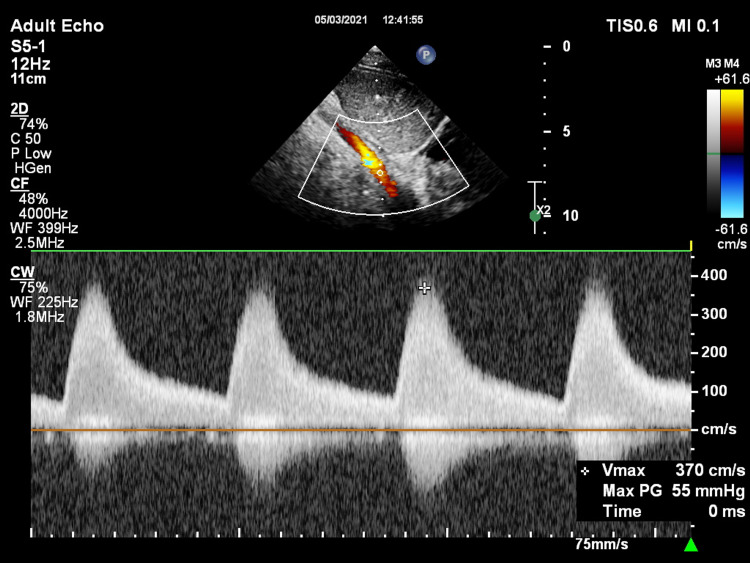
Subcostal view showing turbulent flow in the abdominal aorta with continuous wave Doppler signal showing a peak gradient of 54 mmHg suggesting stenosis. Max PG: maximum pressure gradient; Vmax: maximum velocity
